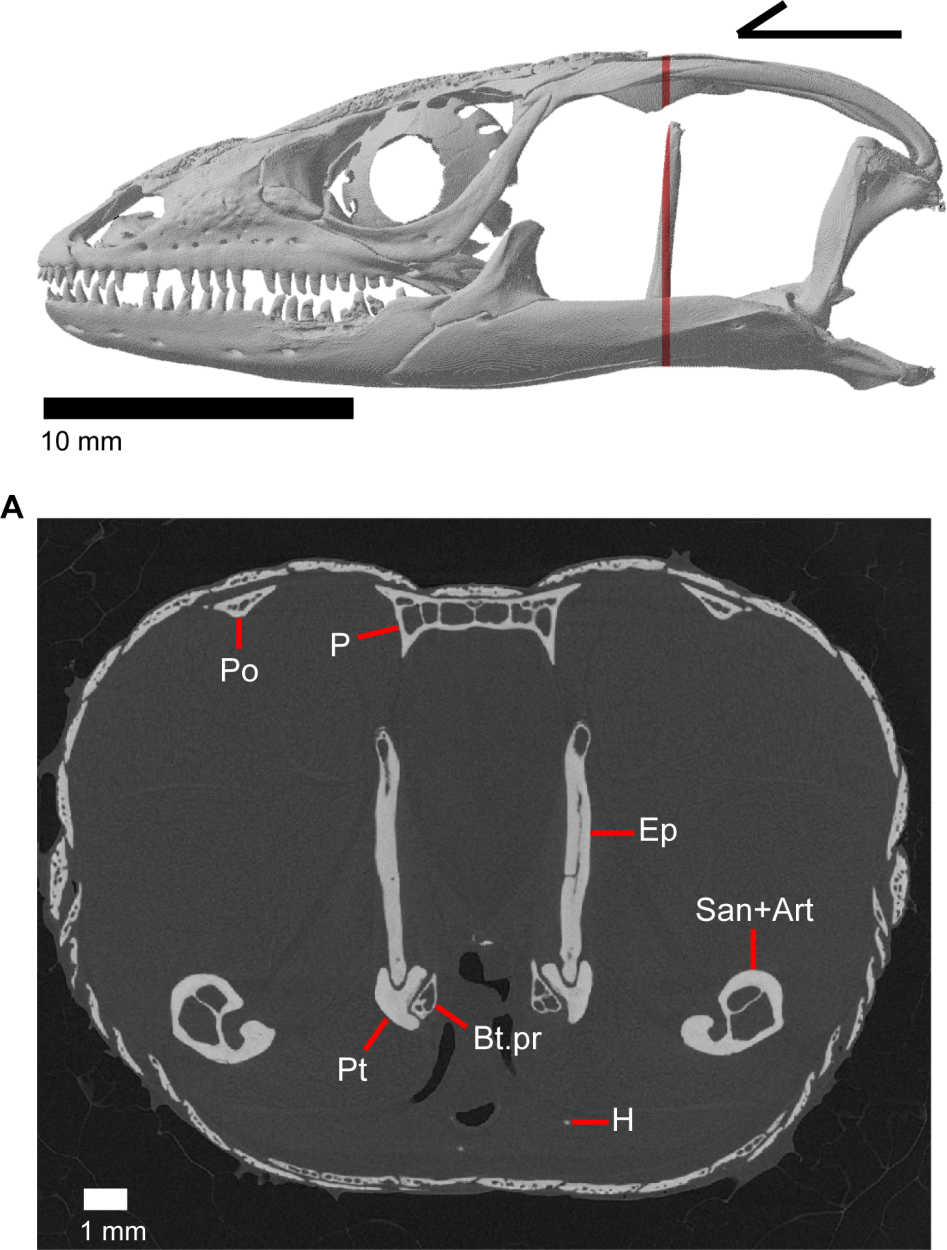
CT coronal slice of *Elgaria panamintina* MVZ 191076.
